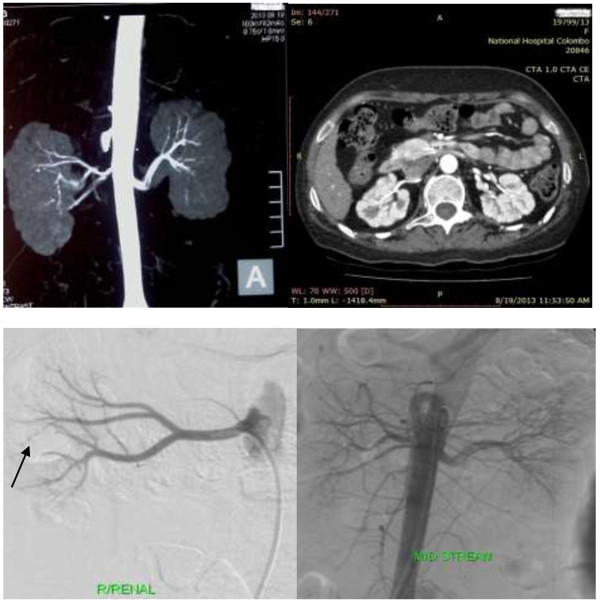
Renal imaging: Row 1: Computed tomography of the abdomen demonstrates structurally abnormal kidneys with irregular contours, suggesting infarction occurring secondary to PAN causing architectural change. Row 2: Digital subtraction angiogram of the kidneys demonstrates early aneurysms on the right renal vasculature.
